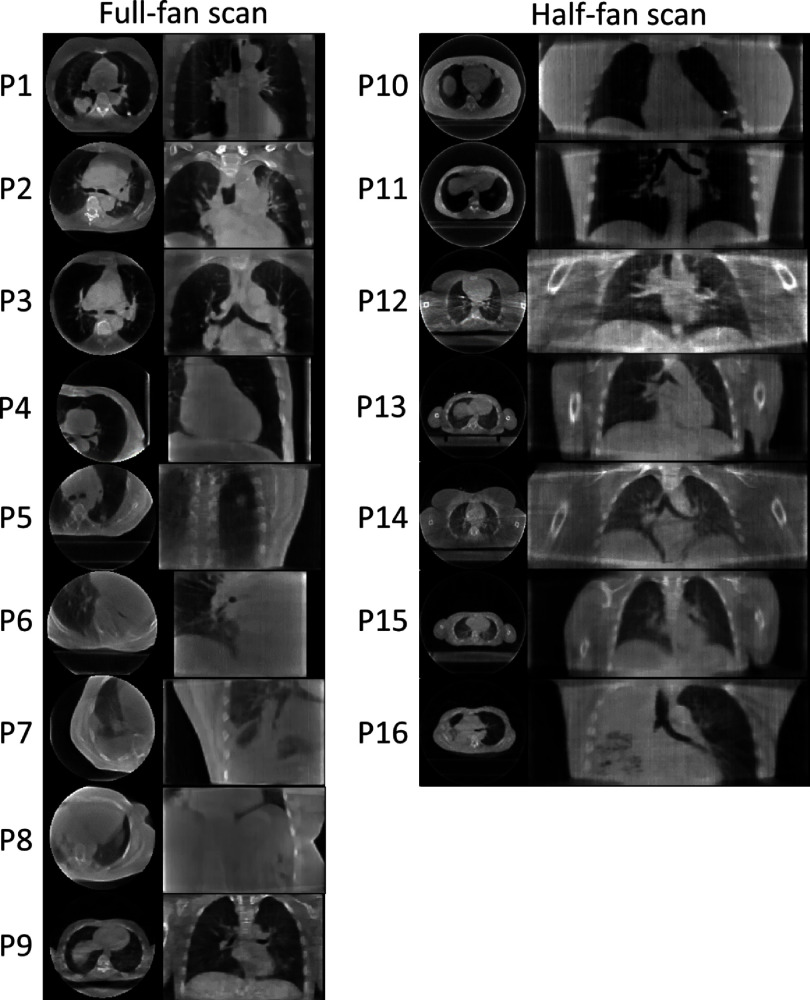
Reference CBCTs reconstructed by DREME for the patient study. Left and right columns present the axial and coronal views of the reference CBCTs for the full-fan and half-fan scans, respectively. Except for P8, whose CBCT scan covered the abdominal region, the other CBCT scans covered the thoracic region.
